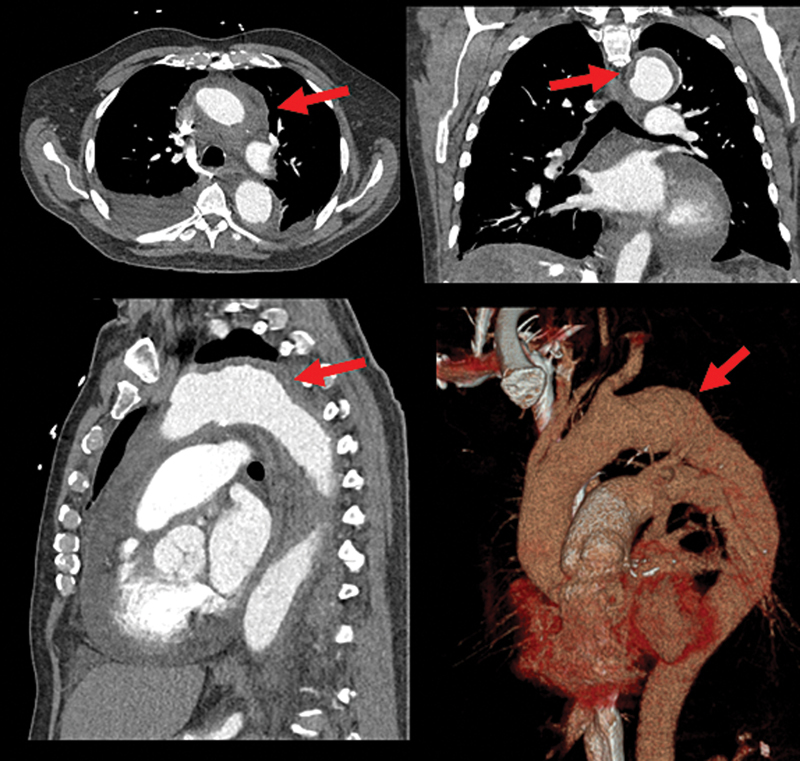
Standard computerized tomography (CT) images, all showing the irregular aortic wall and deposition in periaortic region (arrow).
